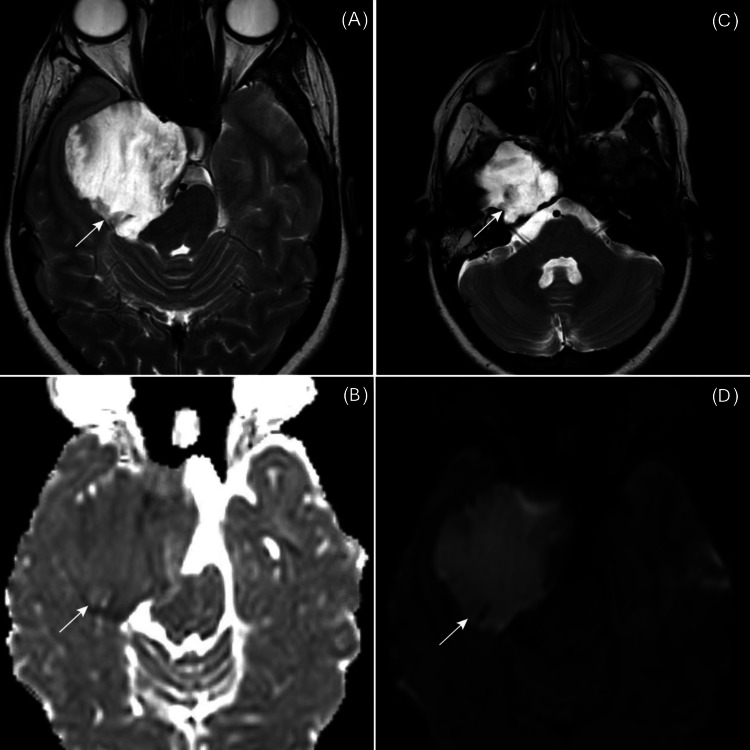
Axial MRI and diffusion-weighted imaging (DWI) of a hyperintense extra-axial lesion at the right petrous apex (A) T2-weighted MRI shows a hyperintense lesion at the right petrous apex with a mass effect on surrounding structures. (B) The ADC (apparent diffusion coefficient) map shows restricted diffusion within the lesion, indicative of high cellularity. (C) T2-weighted MRI highlights the lesion’s extension into the middle cranial fossa, further compressing adjacent areas. (D) DWI confirms restricted diffusion within the lesion, supporting the diagnosis of a tumor.
